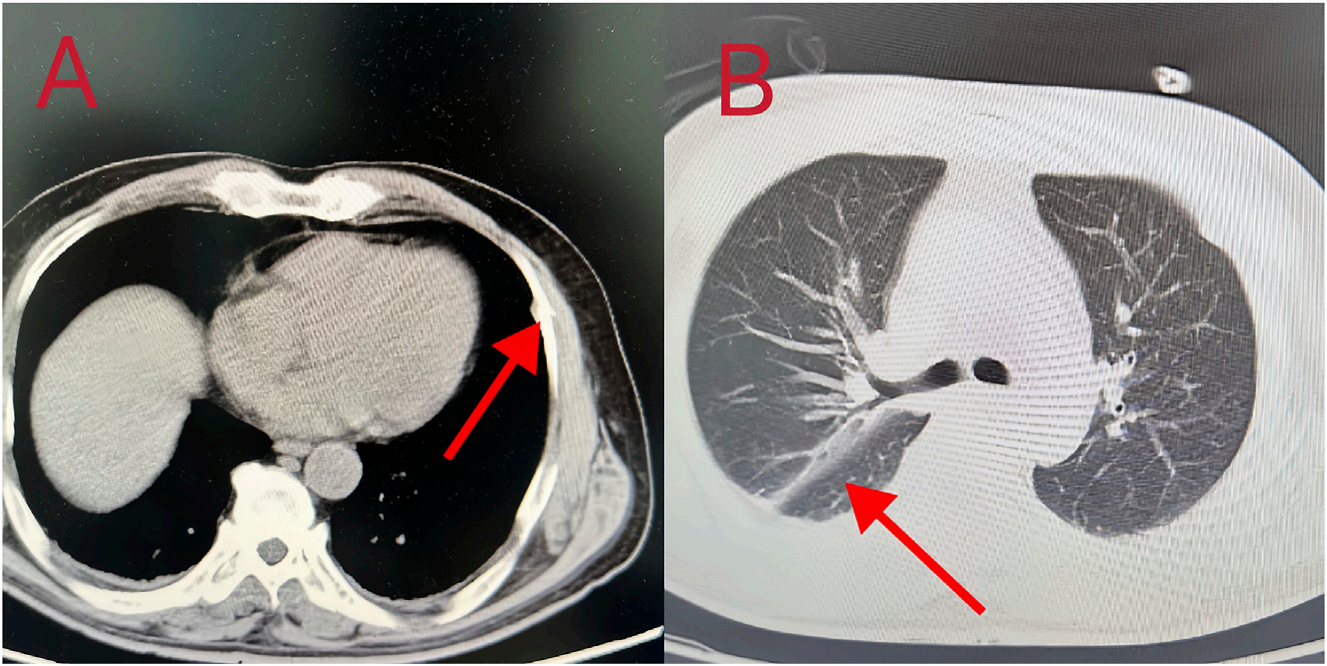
(A, B) Chest CT images showed bilateral pneumonia, a small amount of bilateral pleural effusion, and partial rib bone discontinuity.
